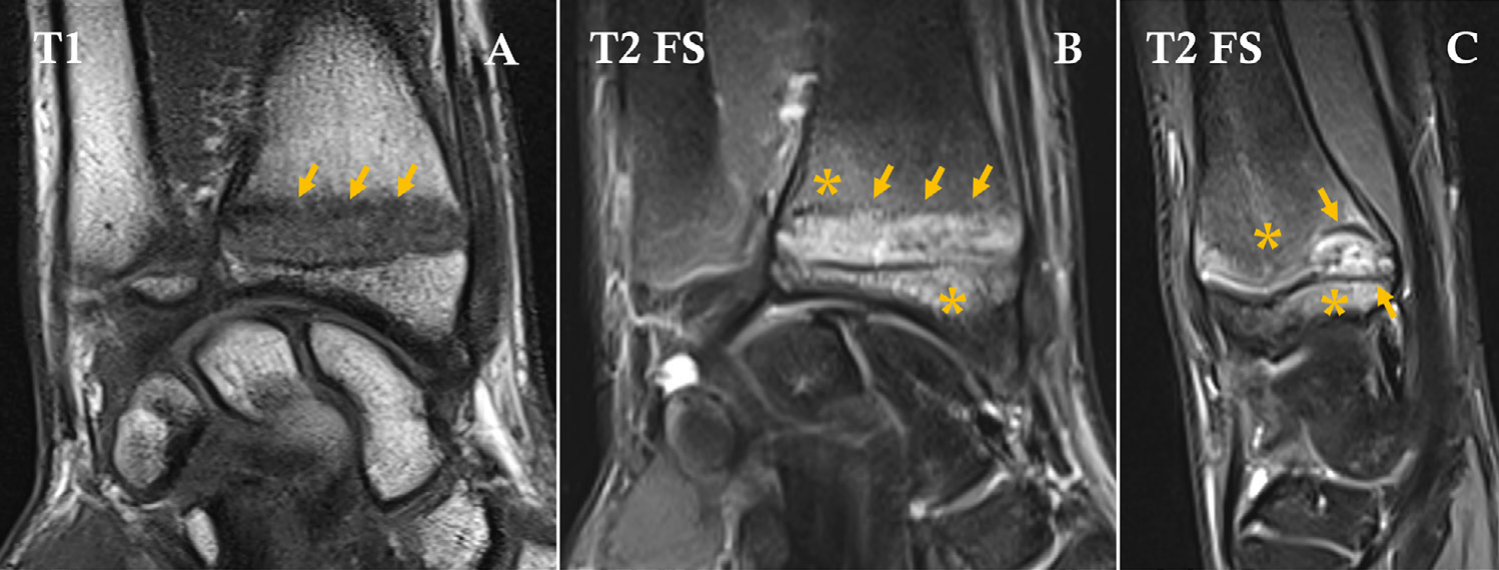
Coronal T1‑weighted (A), coronal and sagittal T2‑weighted fat‑suppressed (B, C) MR images demonstrating focal widening of the volar distal radial physis (arrows) with adjacent metaphyseal and epiphyseal bone marrow edema (asterisks).
